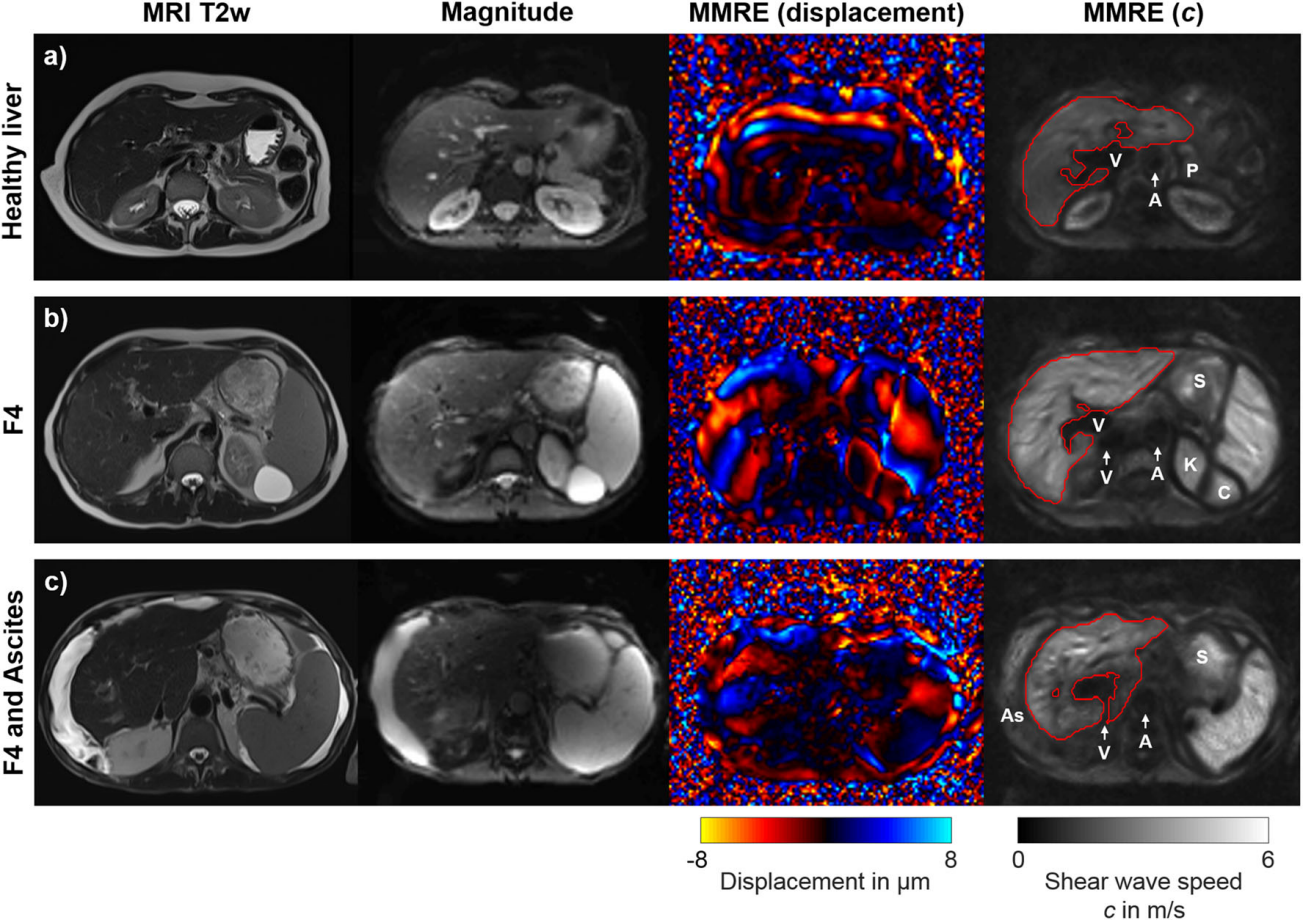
Tomoelastography of the upper abdomen. MRI T2w, conventional T2-weighted image with half-Fourier acquisition single-shot turbo spin echo (HASTE) sequence in axial orientation without contrast medium. Magnitude, morphological magnitude image derived from the multifrequency MR elastography (MMRE) sequence. MMRE (displacement), the shear wave image depicts tissue displacement in and out of the axial plane with red and blue colors. MMRE (*c*), compound multifrequency elastograms represent quantitative maps of shear wave speed (*c*) with bright and dark colors. Besides abdominal organs such as the liver, spleen, and kidneys, these maps also visualize smaller anatomical structures. The region of interest (red) indicates areas included in the analysis. V, hepatic vein/inferior vena cava; A, aorta; P, pancreas; K, kidney; S, stomach; C, kidney cyst; As, ascites. a A 61-year-old female healthy volunteer with mean *c* of 1.67 ± 0.25 m/s. b A 64-year-old male patient with chronic hepatitis C and cirrhosis (F4). Bright colors in the elastogram indicate a pathologically increased mean *c* of 2.59 ± 0.57 m/s. The left kidney (K) and a kidney cyst (C) are distinguishable from the spleen. c A 72-year-old male patient with chronic hepatitis C, cirrhosis (F4), and increased mean *c* of 2.46 ± 0.58 m/s. Shear wave propagation was not hindered by the presence of ascites (As)
